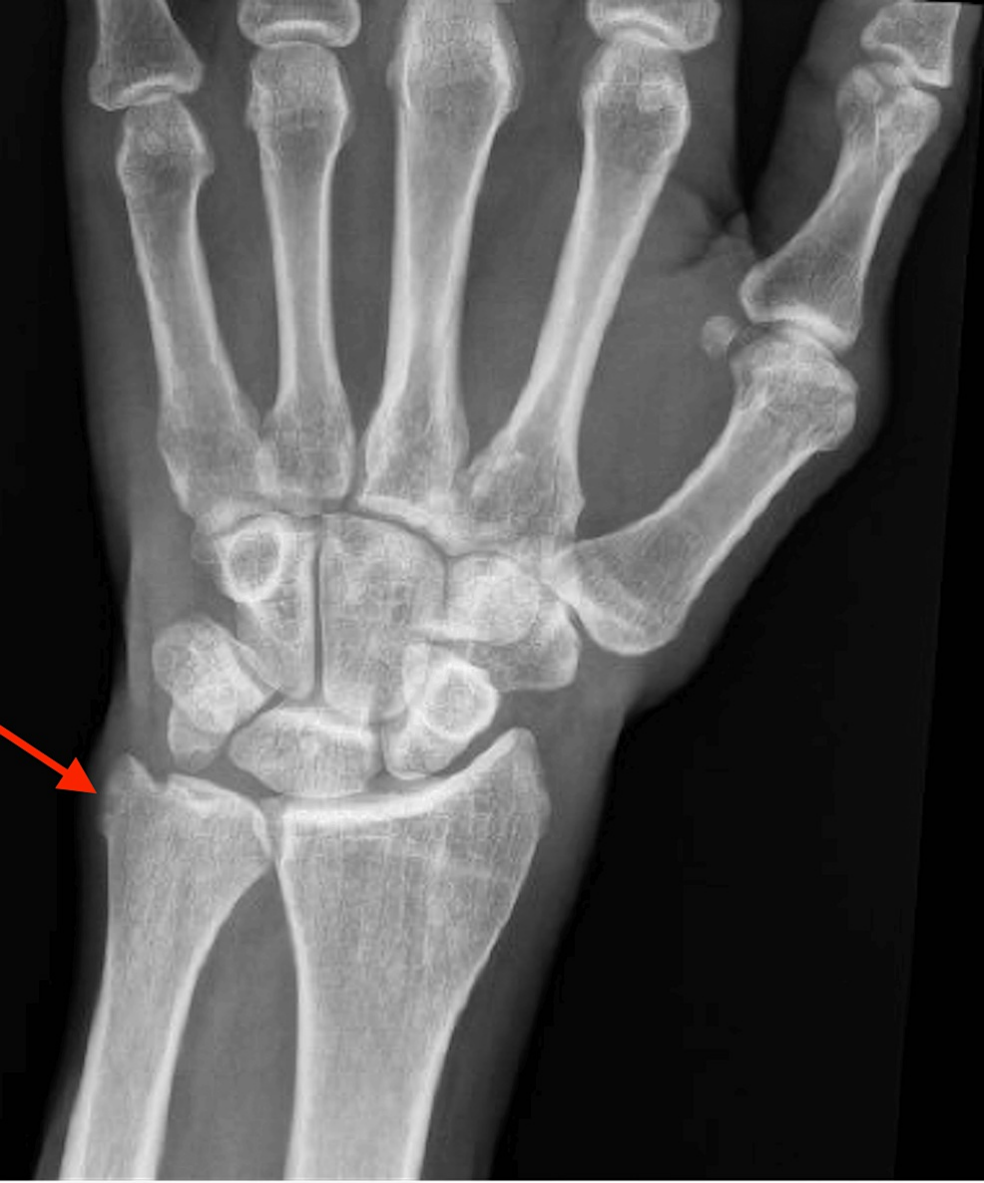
X-ray of the right hand. Red arrow reveals erosion on the ulnar styloid process, significant for inflammatory arthritis
